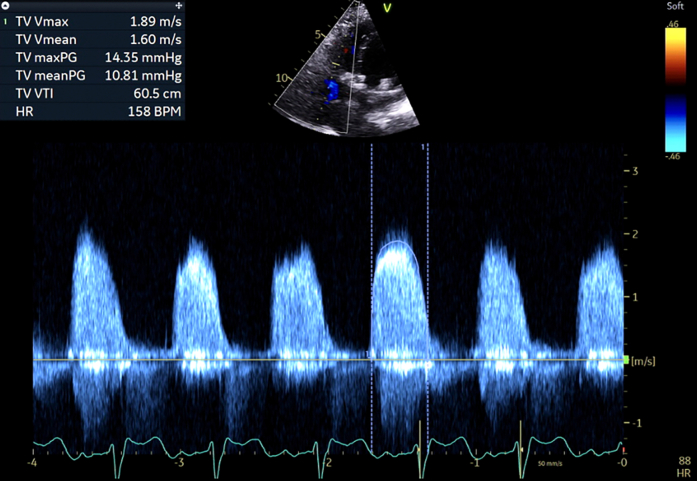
Preprocedural TTE TTE showing a gradient of almost 11 mm Hg across the tricuspid valve. TTE = transthoracic echocardiography.
